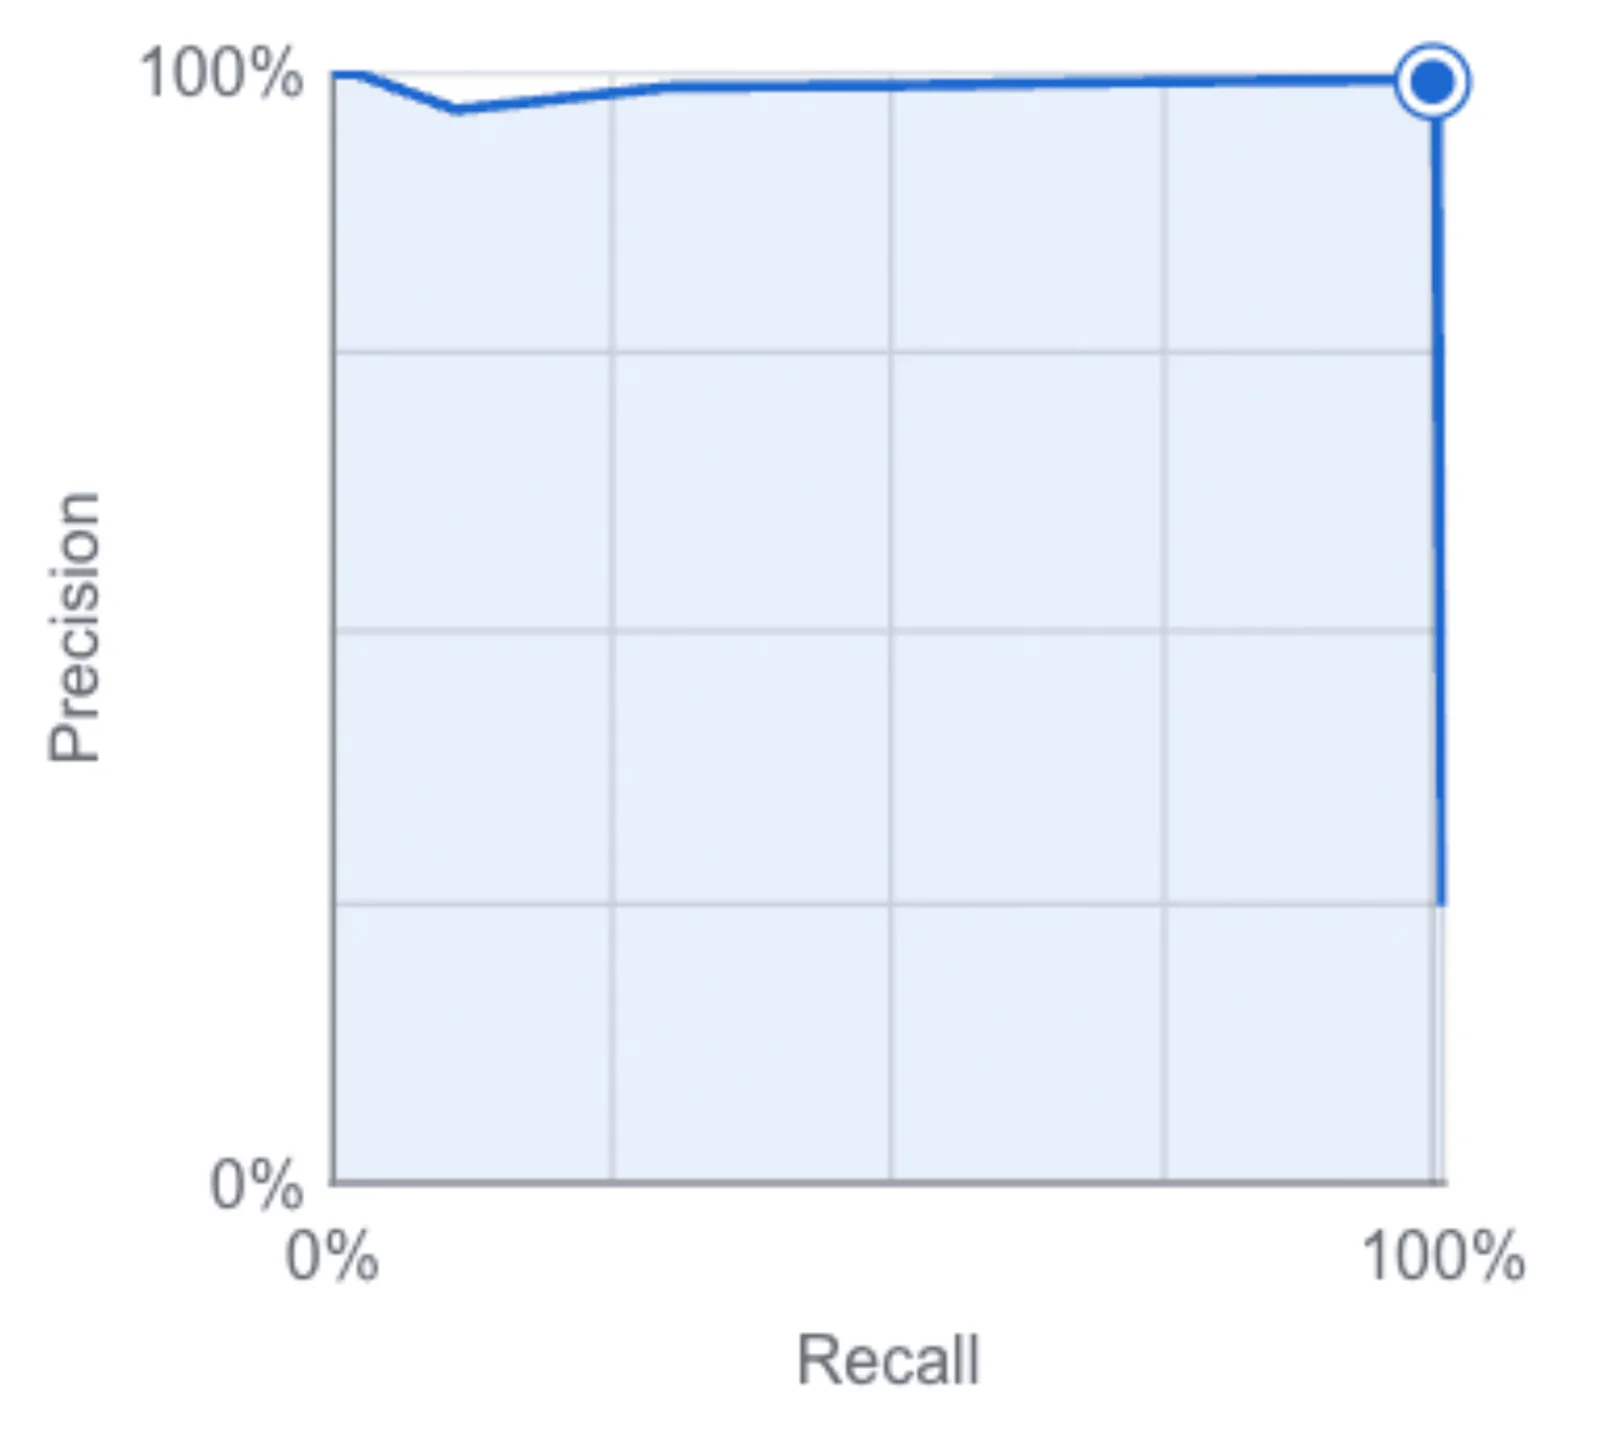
Precision-Recall Curve for the Brain MRI Classification Model The precision-recall curve demonstrates the relationship between precision and recall across different confidence thresholds. The curve remains near the upper-right region of the graph across most thresholds, indicating high precision and recall within the internal test dataset.
